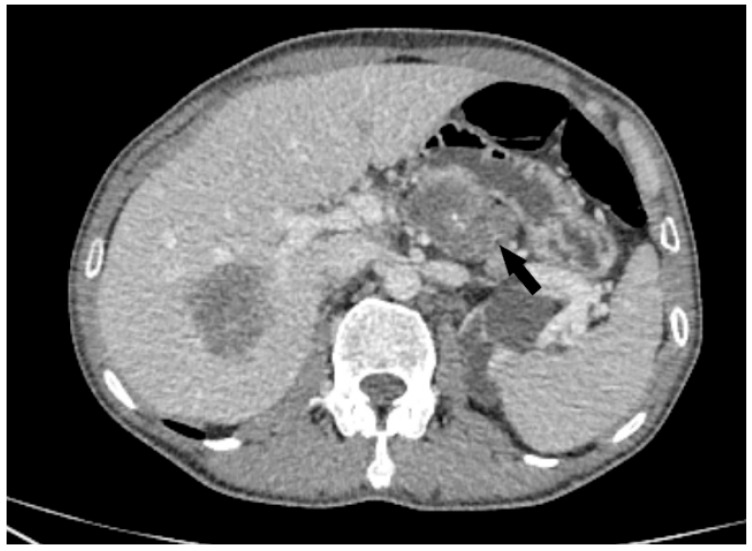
Axial CT image showed a conglomerate of celiac pathological lymph nodes (black arrow) In addition, two solid osteolytic lesions were detected in the sacral and iliac bones on the right, which were highly suspected to be related to the dissemination of the malignant process (Figure 4).
